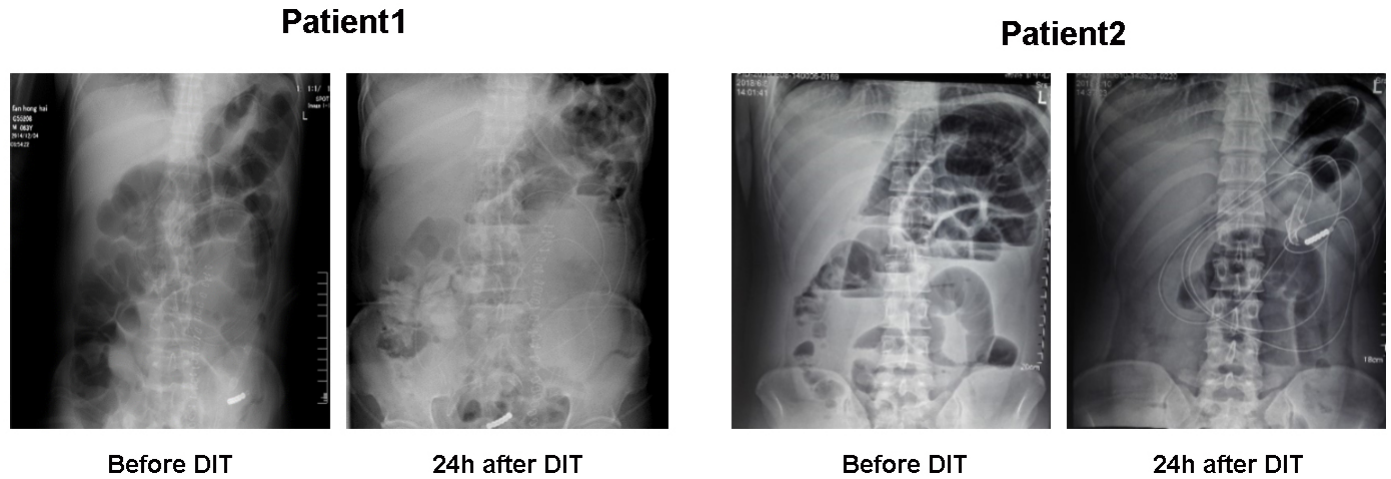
Presentative abdominal X-ray plain films of the patients with acute intestinal obstruction before or 24 h after the deeper intubation technique procedure.
